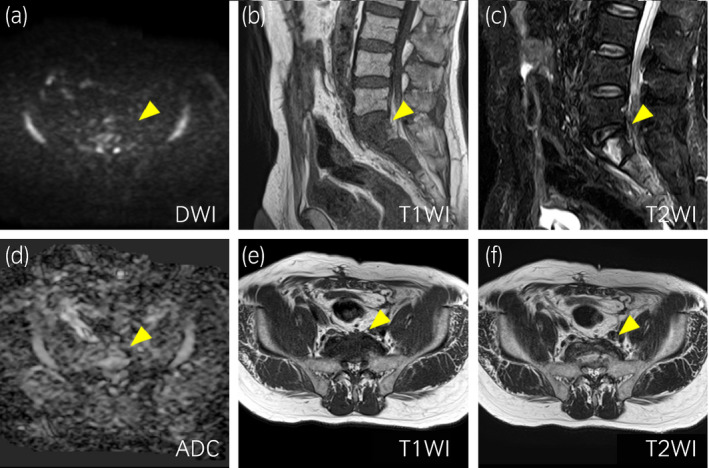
MRI findings when the re‐elevation of HCG levels was observed. (a) DWI, (b) sagittal T1WI, (c) sagittal T2WI, (d) ADC, (e) axial T1WI, (f) axial T2WI.
